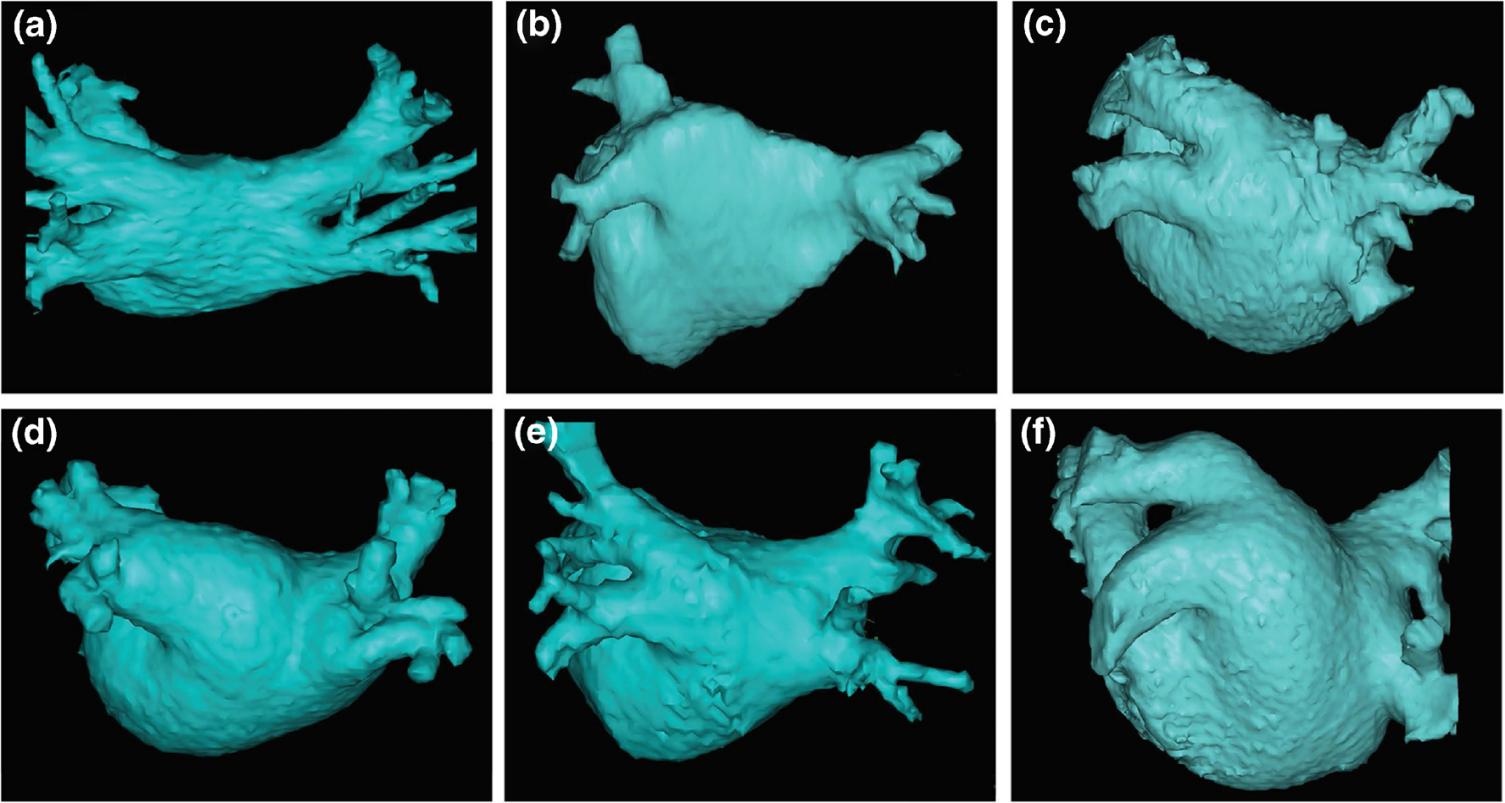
This figure includes six CT or MR images of the left atrium and pulmonary veins viewed from the posterior perspective. Common and uncommon variations in PV anatomy are shown. a Standard PV anatomy with 4 distinct PV ostia. b Variant PV anatomy with a right common and a left common PV. c Variant PV anatomy with a left common PV with a short trunk and an anomolous PV arising from the right posterior left atrial wall. d and e Variant PV anatomy with a common left PV with a long trunk. f Variant PV anatomy with a massive left common PV
